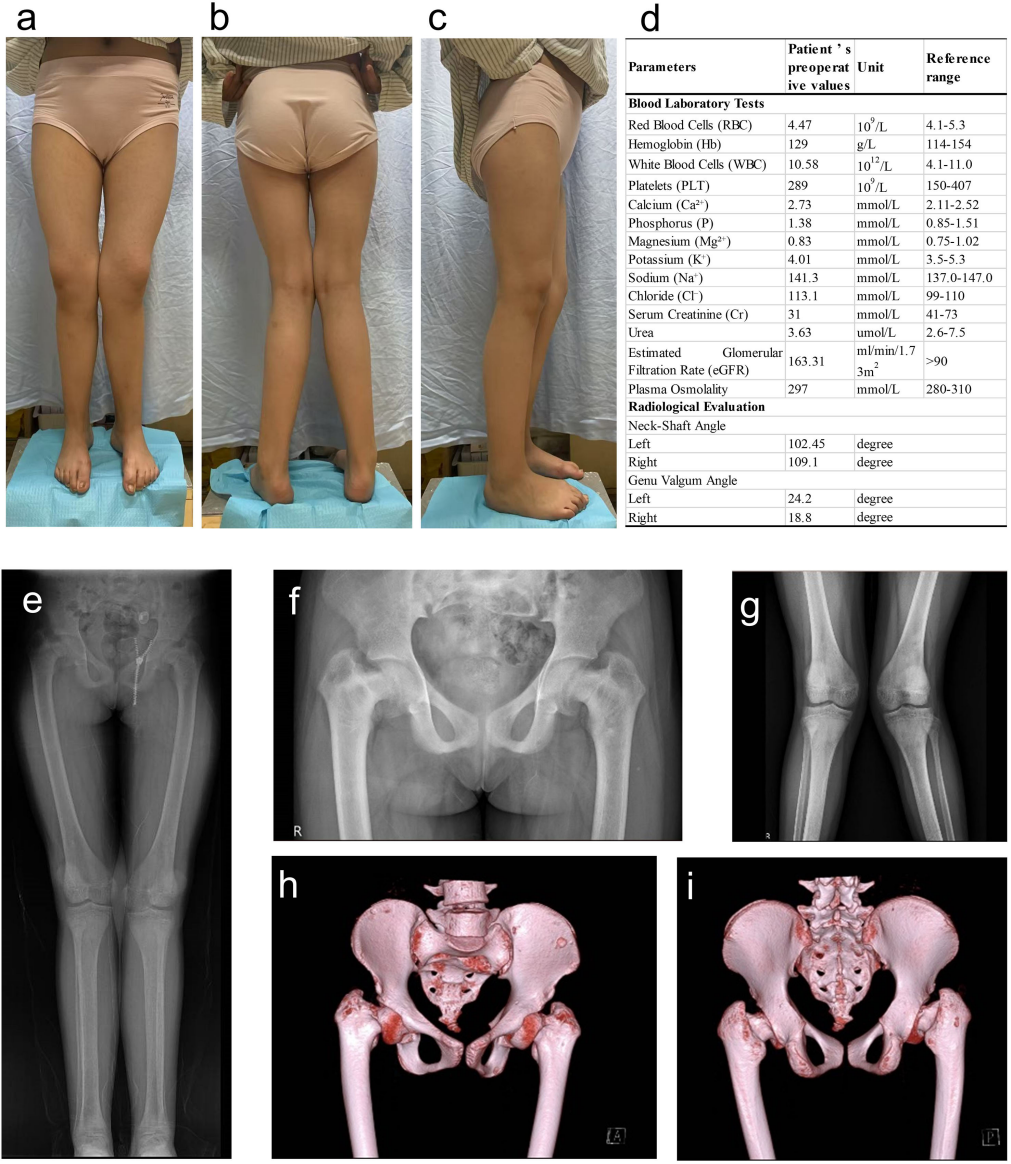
Clinical Findings at admission. (a–c) Clinical photographs (anterior, posterior, and lateral views) showing bilateral coxa vara and genu valgus. (d) Laboratory tests and imaging results; (e) Full-length lower limb radiograph. (f, g) Anteroposterior (AP) radiographs of the hip and knee joints. (h, i) 3D CT reconstruction of the hip joints.
